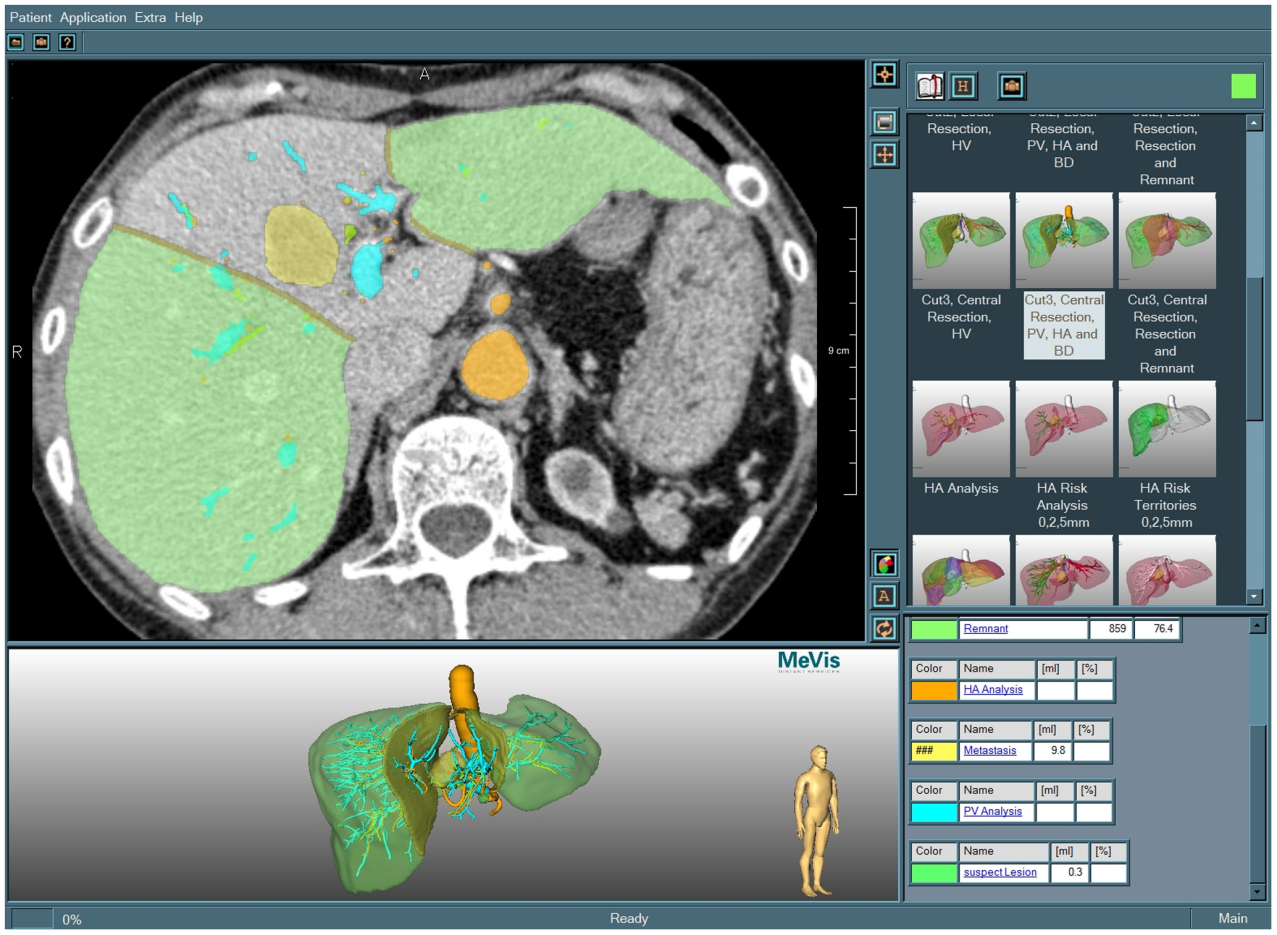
Screenshot of the LiverViewer. The user interface is divided into four parts: CT slices with color overlays (top left), interactive surface shaded objects (bottom left), resection proposal selection (top right) and analysis data (bottom right).
